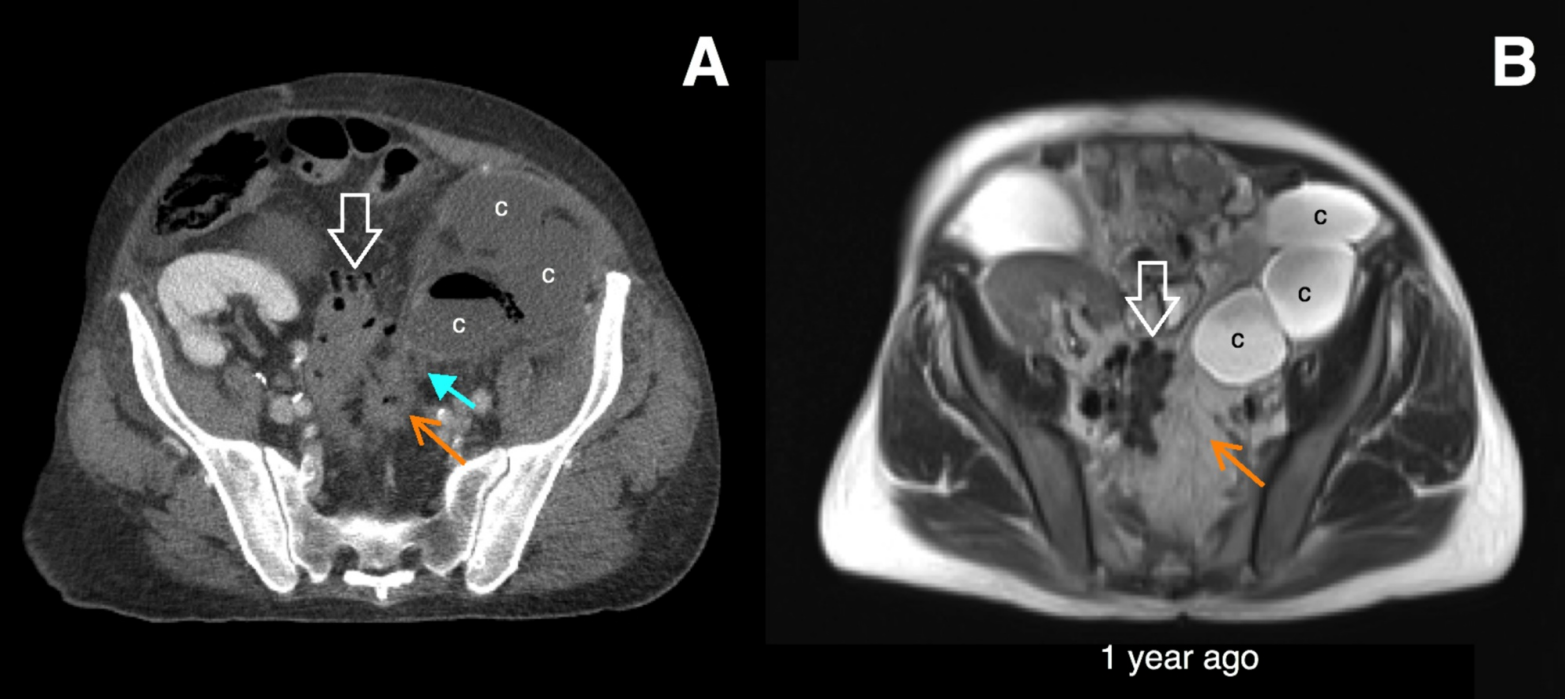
Initial imaging of the cyst infection. Axial computed tomography with intravenous contrast (A) and T2 HASTE magnetic resonance imaging (MRI) (B) show sigmoid diverticulosis (open white arrows) and multiple lower pole renal cysts (c). There is an inflamed diverticulum which is new compared to the prior MRI (orange arrows). Inflammatory stranding is contiguous between the diverticulum and a gas-containing lower pole cyst, which was highly concerning for a renocolic fistula. Right lower quadrant transplant kidney is also seen.
